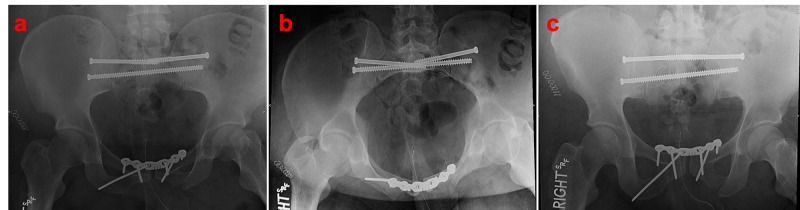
Postoperative AP (a), inlet (b), and outlet (c) radiographs demonstrating successful anterior and posterior pelvic ring fixation. AP: anteroposterior
